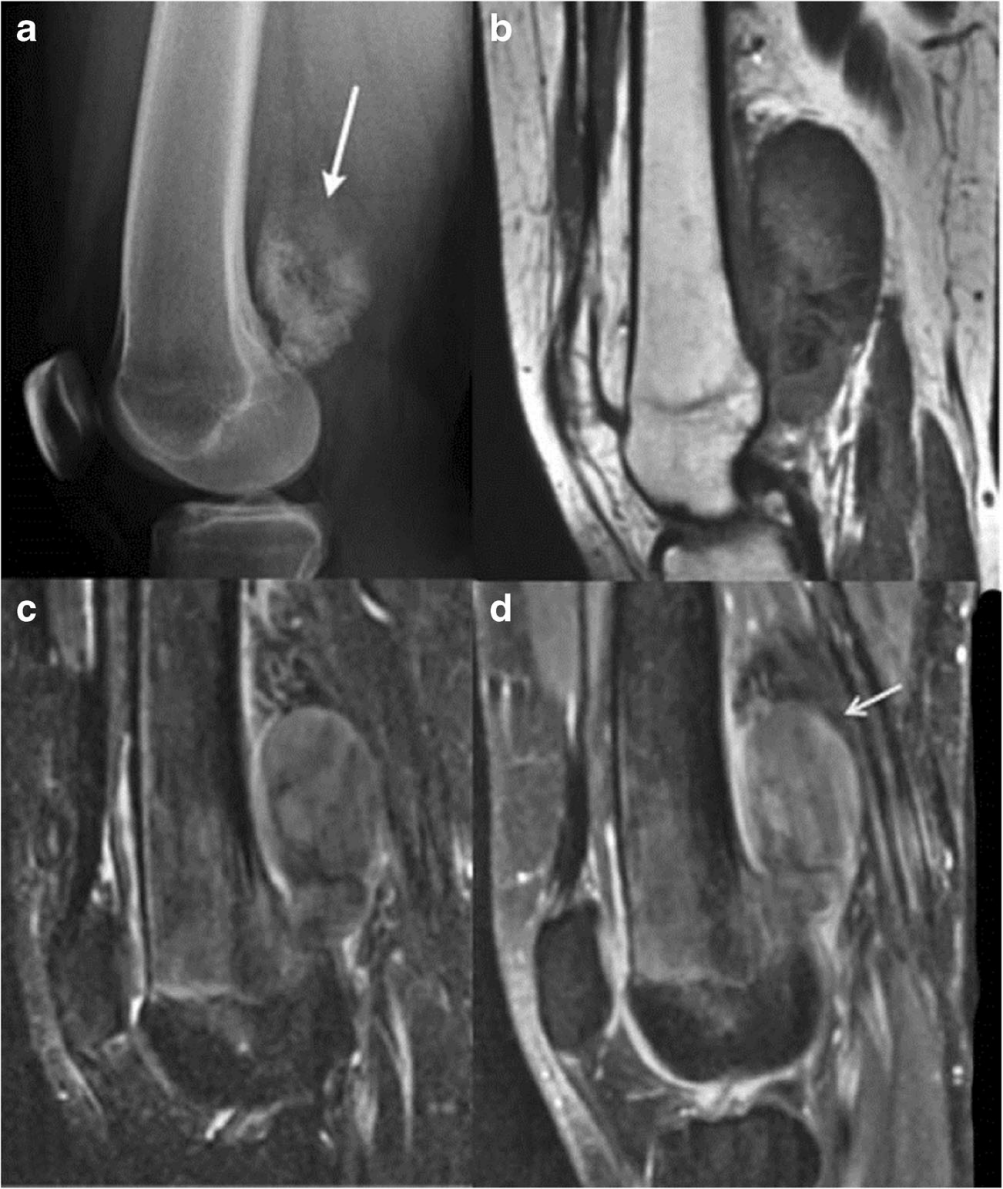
Parosteal osteosarcoma on the left distal posterior femur. A lateral radiograph of the left knee a shows a large exophytic mass with central dense ossifications adjacent to the femur (*arrow*). Sagittal T1 b and T2 c MR images demonstrate heterogeneous signal through this large soft-tissue tumor on both T1 and T2. A sagittal T1 post-contrast image d reveals heterogeneous contrast enhancement (*arrow*)
